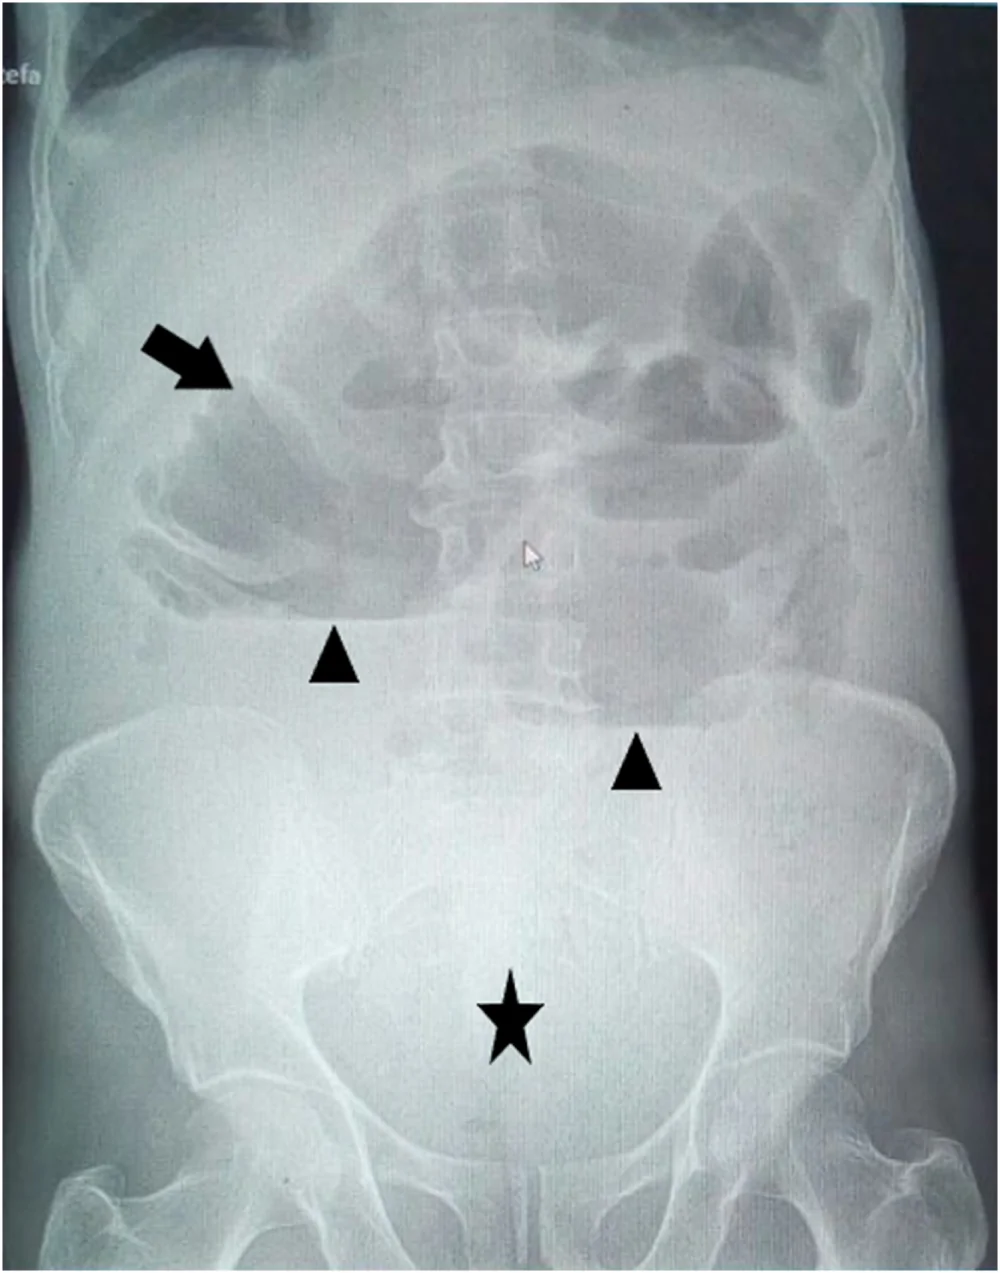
Erect anteroposterior (AP) abdominal radiograph demonstrating findings suggestive of cecal volvulus. The solid black arrow indicates the massively distended, ectopic cecum displacing into the mid-to-left upper abdomen with notable preservation of the haustral folds. The black arrowheads point to prominent, distinct air-fluid levels within the dependent portions of the obstructed bowel loops, and rectal gas paucity is indicated by the black star.
